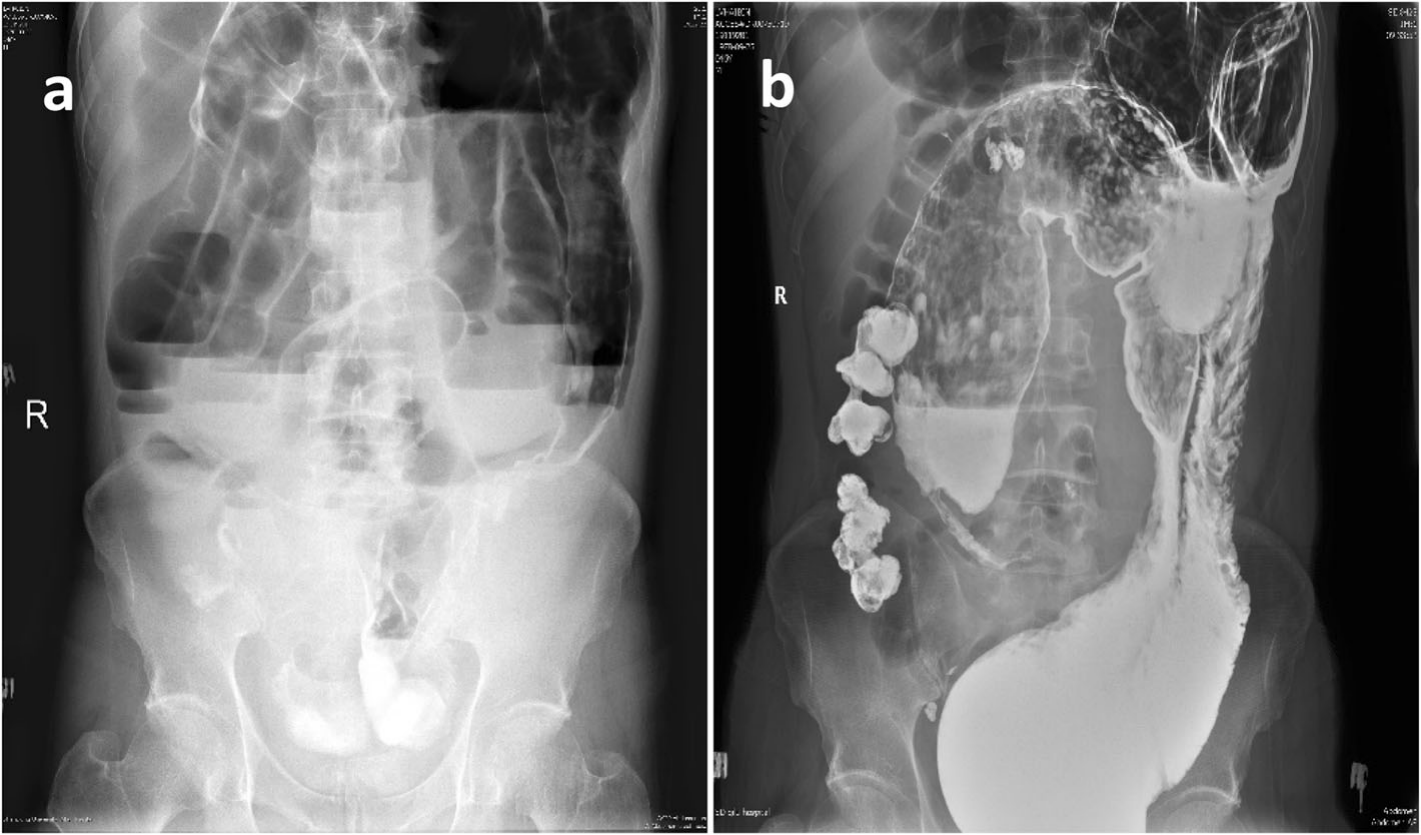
a-b. Single anteroposterior abdominal radiograph showing markedly dilated colon in the initial stage
